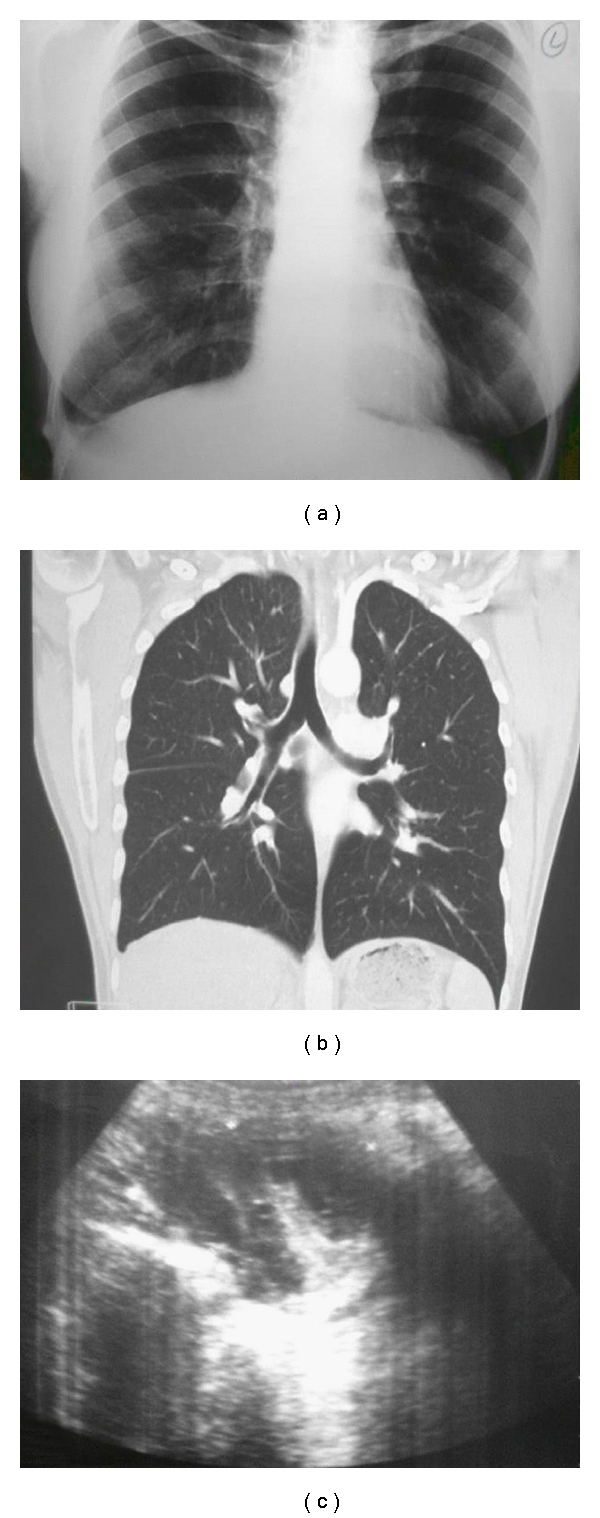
(a) Chest radiography showed a closed right-sided costaphrenic angle and minimal pleural effusion. (b) CT scan did not demonstrate any abnormality. (c) Pelvic US revealed a left adnexal semisolid mass, with a thick wall and septa.
